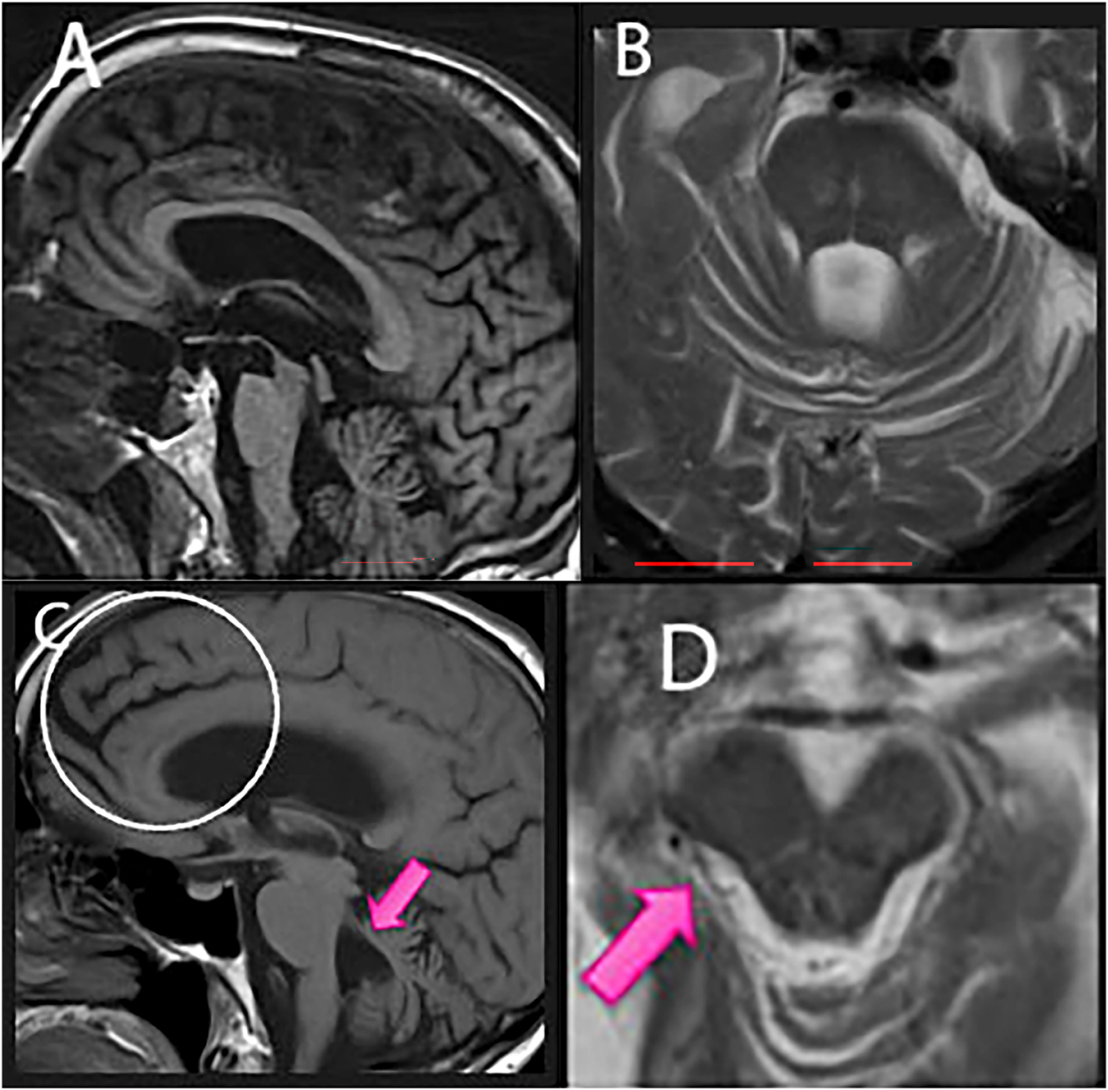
81-year-old male with atypical PD, tremor, loss of balance, dementia and vertical gaze difficulty. T1-weighted sagittal image (A) shows profound midbrain tegmental atrophy, manifesting a hummingbird sign. T2-weighted axial (B) image and T1-weighted sagittal image off-midline (C) show atrophy in the bilateral superior cerebellar peduncle and frontal dominant volume loss (circle). A magnified T2-weighted axial image (D) shows concavity along the posterior lateral midbrain (pink arrow), consistent with the morning glory sign. The findings are consistent with progressive supranuclear palsy (PSP)
